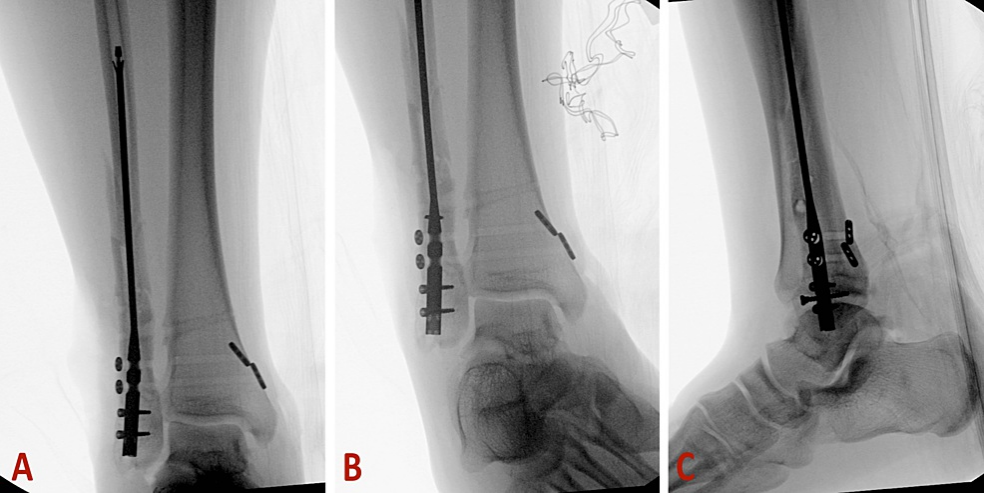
Intraoperative radiographs depicting placement of the two suture constructs through the fibular nail. A: Anterior-posterior view. B: Oblique view. C: Lateral view
